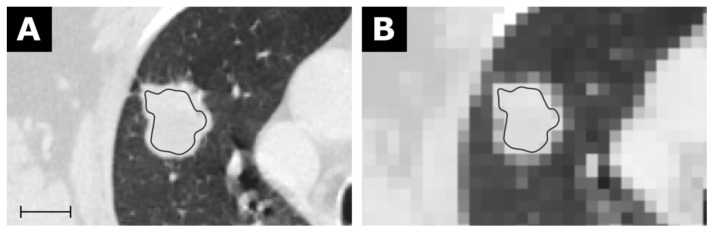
CT of a bronchial carcinoma (A) with a corresponding map for the feature Mean with a resolution of 5 × 5 × 5 mm in (B). Scale indicates 2 cm. The value for Mean from the conventional extraction was 24.9 and 22.2 for the map. Details are available in electronic Table S2.
